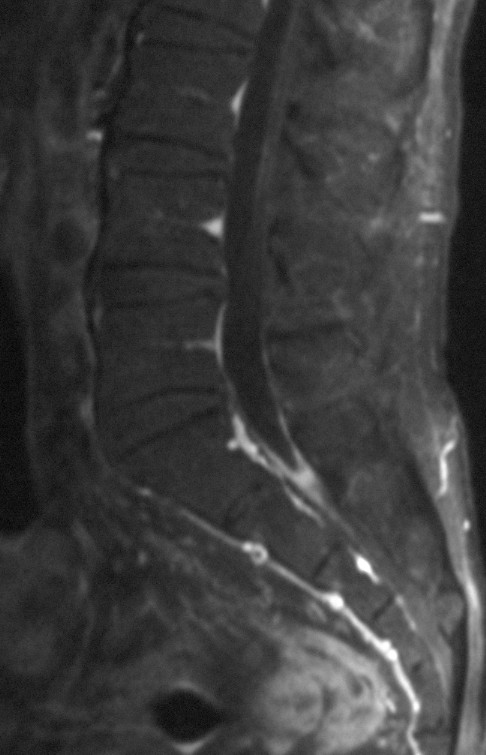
Sagittal section of Gadolinium-enhanced T1-weighted MRI revealed a V-shaped caudal dural sac of the sacral spine along the sacral dura mater.
